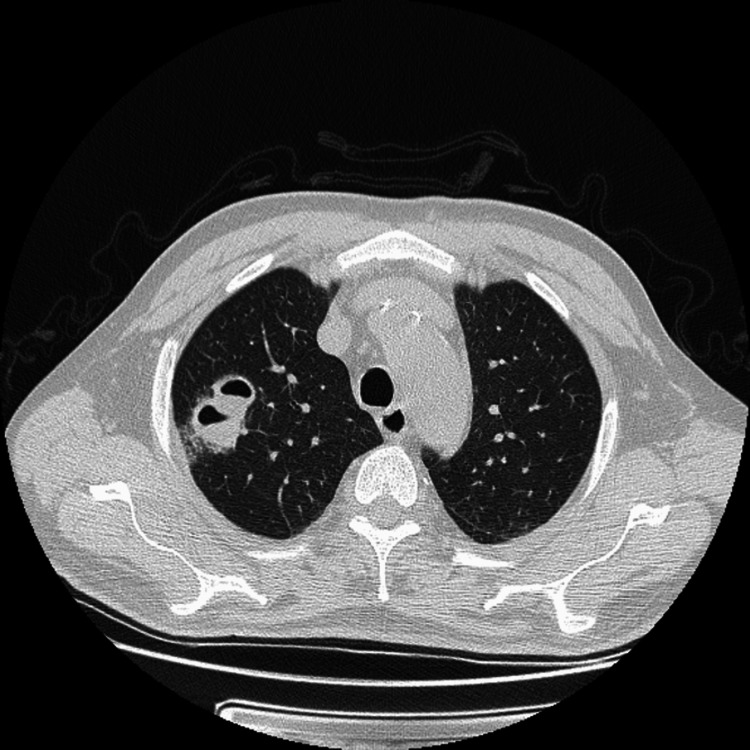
A thoracic CT scan revealed bilateral pulmonary cavitations at the superior lobes
